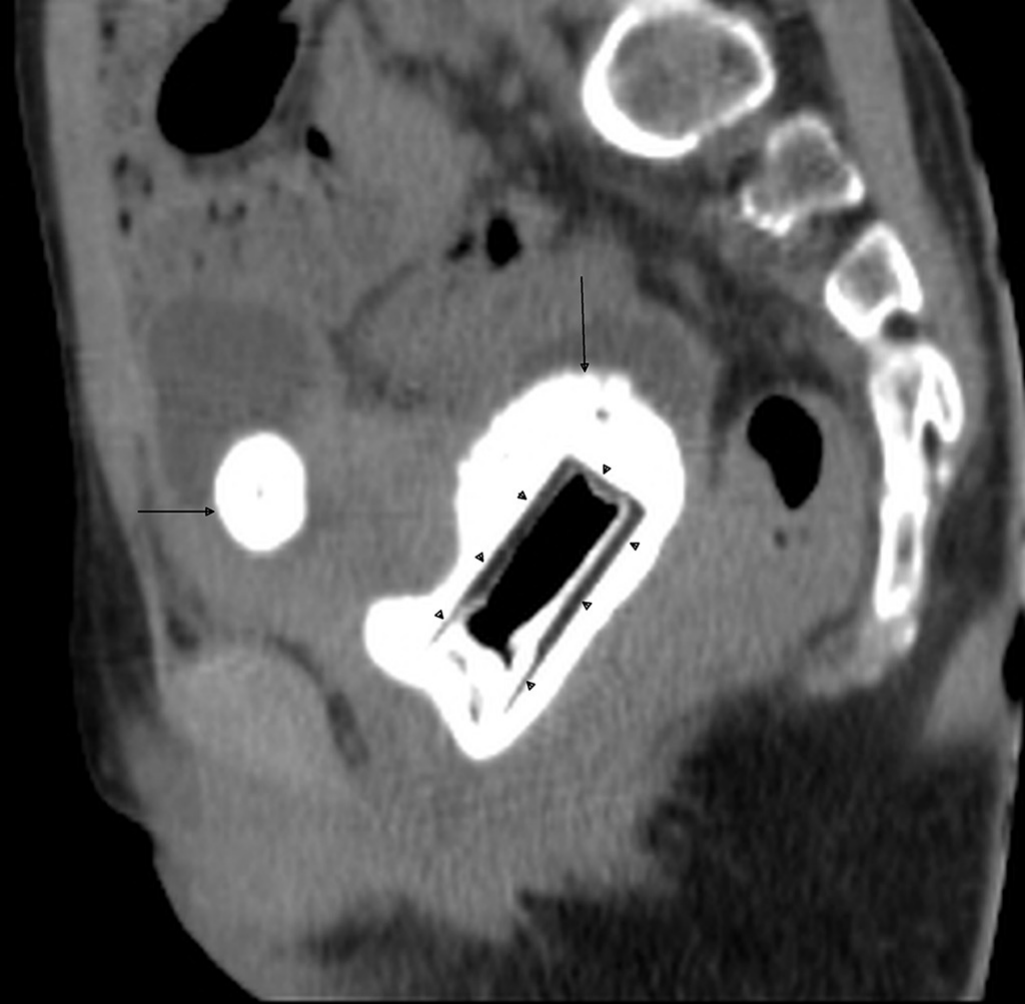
Sagittal reformatted non-contrast CT scan of the pelvis showing a big vaginolith (vertical arrow) surrounding the foreign body (arrowheads). Vesical calculus (horizontal arrow) is also seen in the lumen of the urinary bladder.
